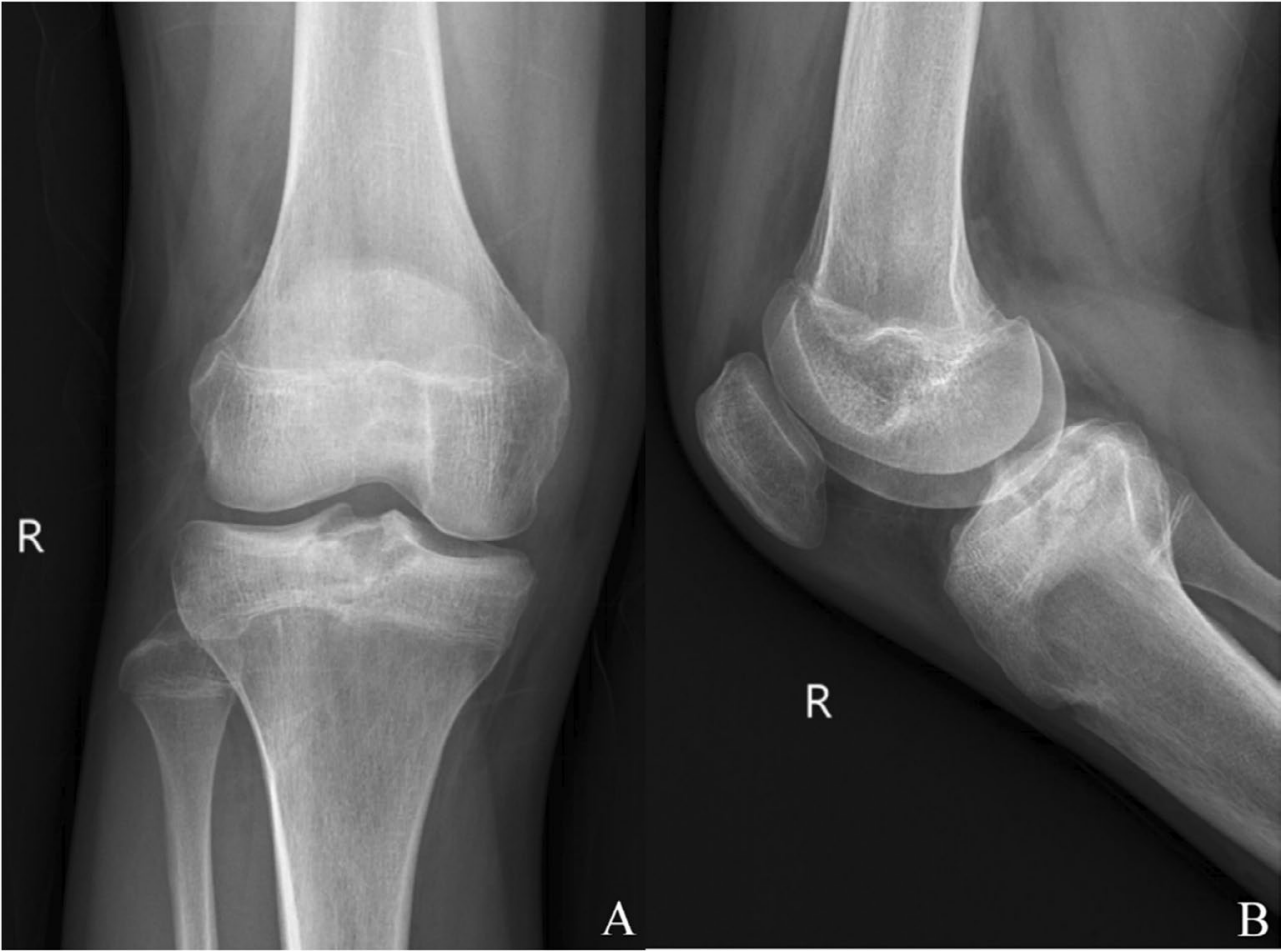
Radiographs of right knee showing a good osteointegration of the bone graft 3 months after surgery: anteroposterior (A) and lateral (B) views.
